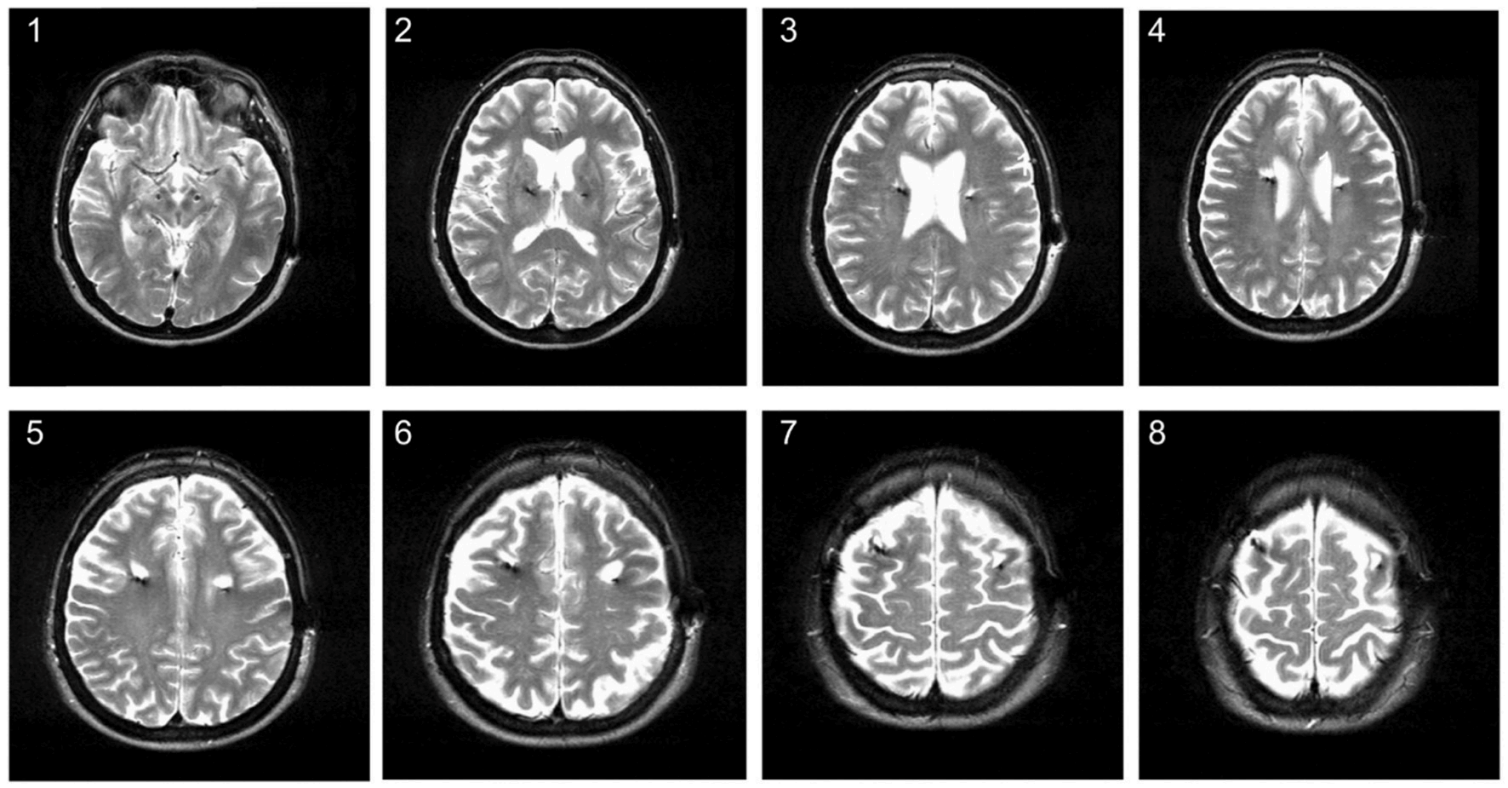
Hyperintense signal changes on MRI in a 58-year old patient treated with DBS for Parkinson's disease. The MRI was conducted about 3 years after STN-DBS surgery. The image shows hyperintense white matter lesions around the upper electrode lead on axial T2 series.
